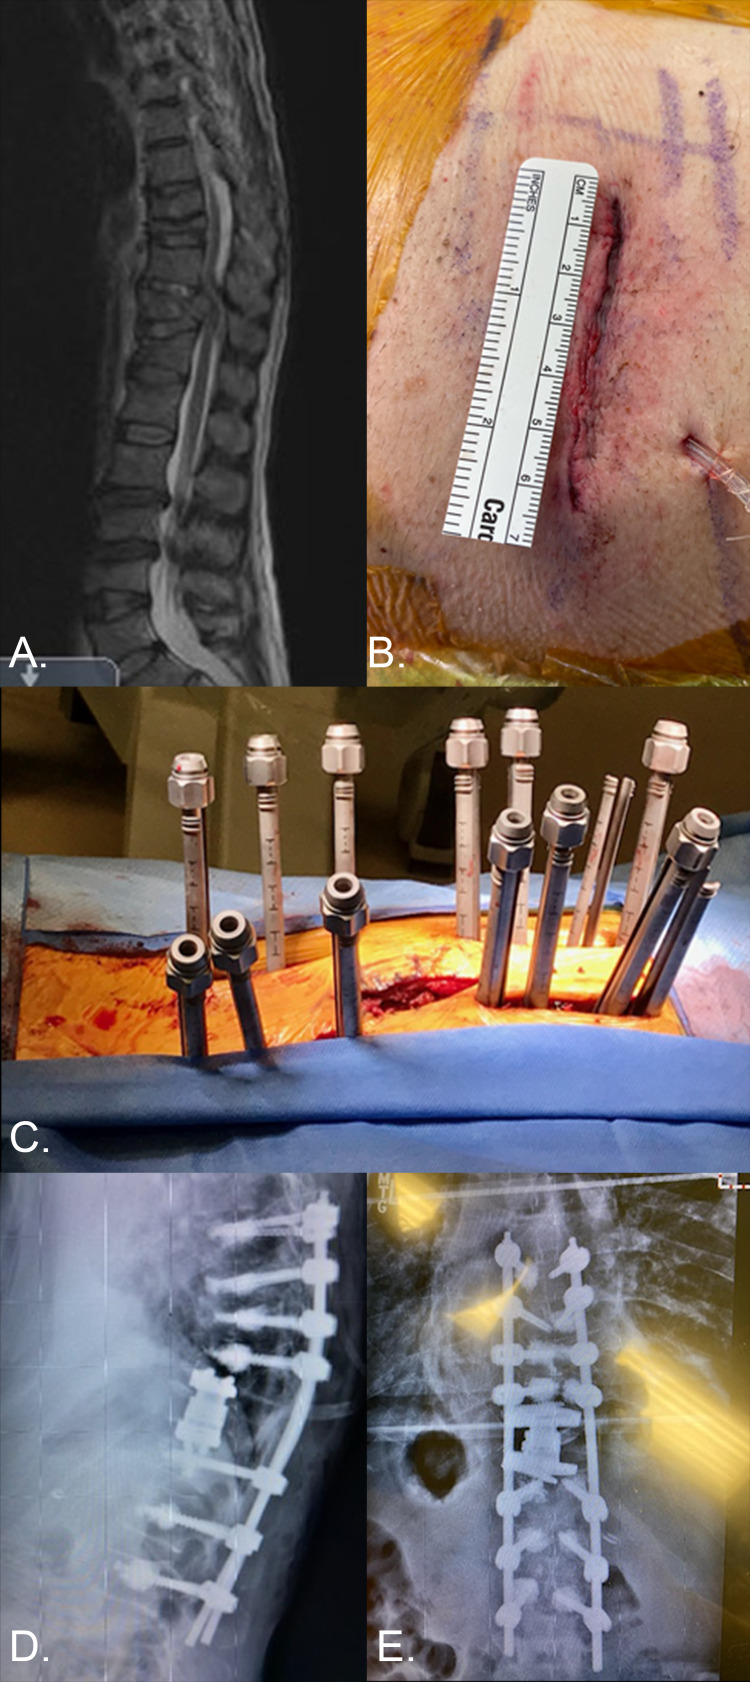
AR case by CAM employing the xvision platform. A. T2W MRI demonstrating T11 pathologic compression fracture resulting in severe spinal canal compression, as well as multiple chronic compression fractures in a 58-year-old myelopathic male with history of lung cancer and severe osteoporosis (T-score:-2.8). B. Stage 1 mini-open retropleural thoracotomy for T11 corpectomy. C,D,E: Stage 2 percutaneous cement augmented T7 - L2 pedicle screw and rod fixation. Case highlights the utility of AR applications in executing technically demanding MIS applications. In this case, the precise insertion of thoracolumbar pedicle screws via AR permitted safe cement augmentation without visceral, vascular, canal, or foraminal cement extravasation. CAM: Camilo A. Molina.
